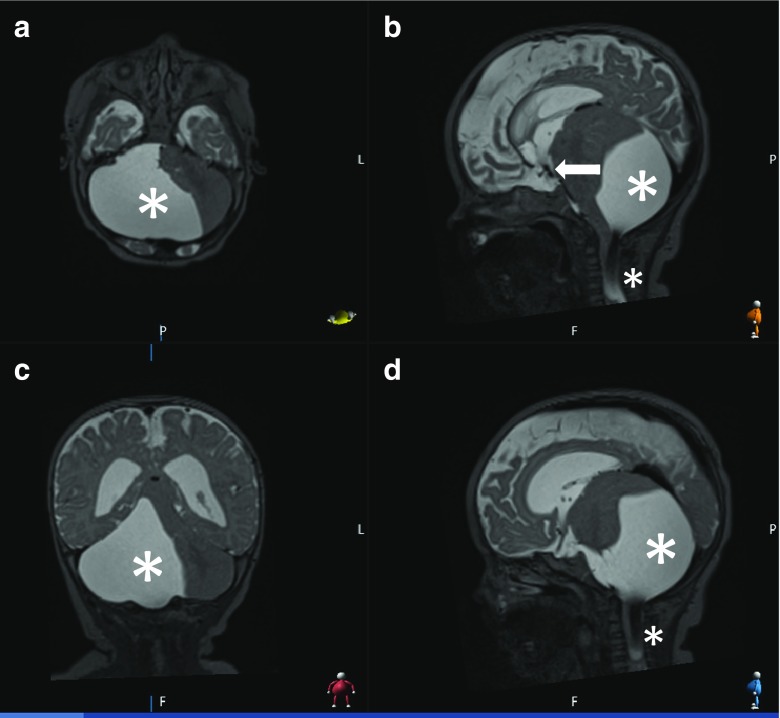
T2-weighted MRI demonstrating the increase in size of the AC with extension into the craniocervical junction (asterisks) with a functioning ventriculostomy (arrow); axial a; sagittal b; coronal c; paramedian sagittal d
